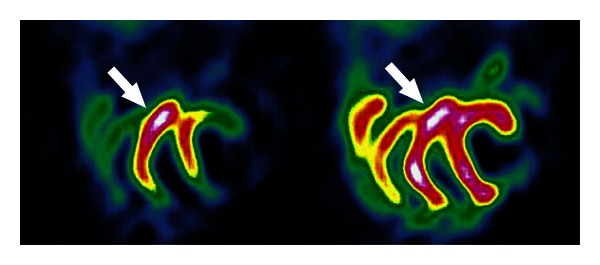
The same patient, abdomen SPECT, coronal slices. Massive leukocyte uptake in the whole small intestine—m. Crohn.
